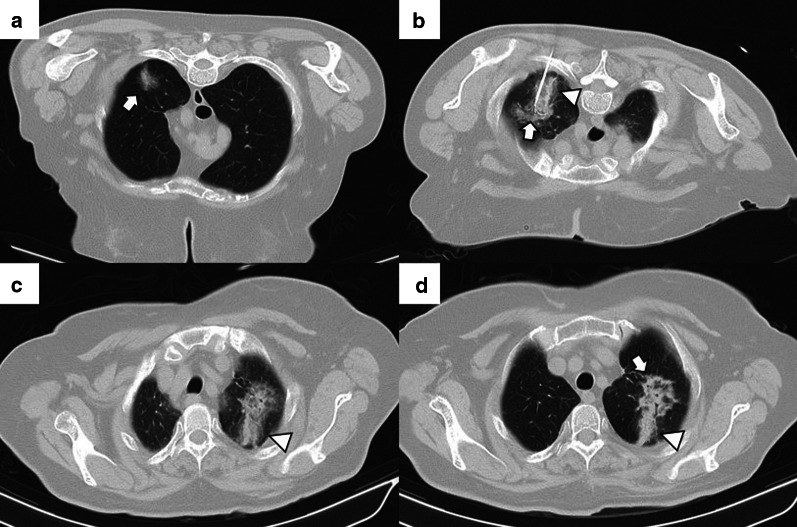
Mild lung hemorrhage in the path of the ablation probe. a, b CT-guided percutaneous RFA with an expandable probe of a stage 1 NSCLC (arrow in a). The expandable probe completely covers the tumor in b (arrow), and the formation of a lung hemorrhage is visible in the probe’s path (triangle). c In the CT scan performed immediately after the procedure, we can observe a mild lung hemorrhage related to the probe’s path (triangle). d The lung hemorrhage remains stable in the CT 24 h after the procedure, and the patient remains asymptomatic. Note the presence of a ground-glass halo englobing a consolidative center, a suggestive sign of a correct ablative treatment (arrow in d)
